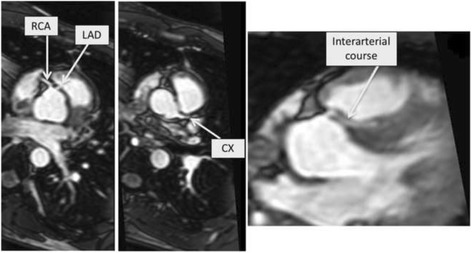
Cardiovascular magnetic resonance imaging showing interaterial course of left anterior descending artery. Cardiovascular magnetic resonance imaging showing the course of the anomalous left anterior descending coronary artery (LAD) arising from a separate ostium of the right sinus of Valsalva with a potentially malignant interarterial course, in which the LAD passes between the aorta and pulmonary trunk. The left circumflex (LCX) artery is located in its usual expected position at the left sinus of Valsalva
